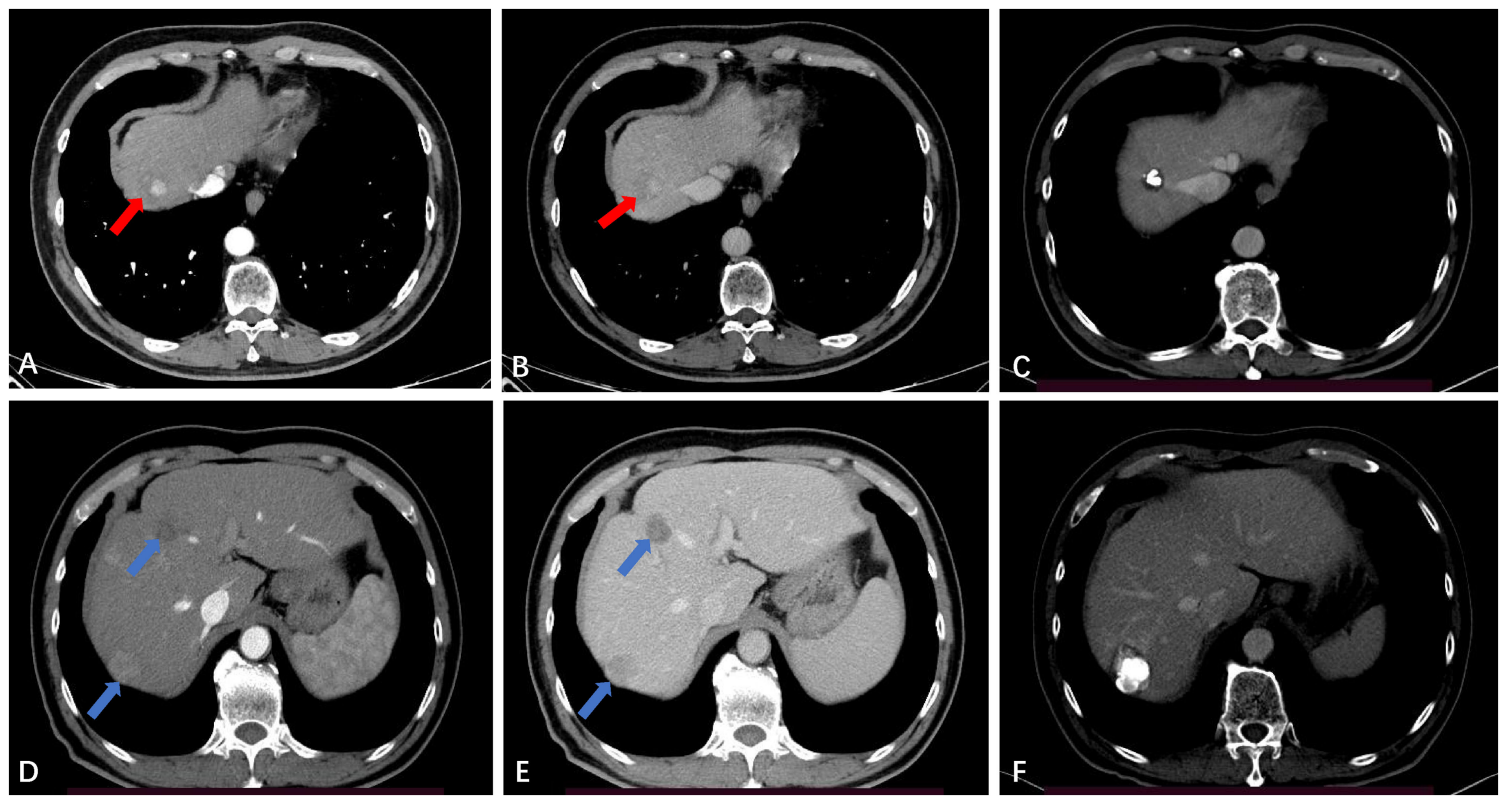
Intrahepatic distant recurrence (IDR) after TACE-RFA for HCC in a 56-year-old man. (A, B) Dynamic contrast-enhanced axial CT scan shows a single HCC with diameter of 2.4cm (red arrow). (C) Axial CT scan shows the complete ablation zone, with “intratumoral lipiodol deposition” visible in the ablation zone. (D, E) CT scan obtained during the hepatic arterial phase and portal venous phase 51 months after TACE-RFA shows IDR (blue arrows), with the largest lesions measuring about 2.7 cm. The two lesions received RFA and TACE-RFA, respectively. (F) Iodide oil deposits are visible in the largest lesion.
